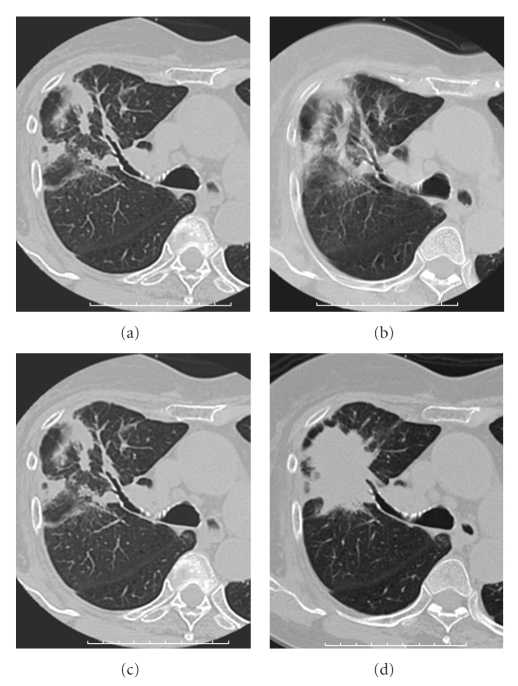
Serial CT images after SBRT. (a), (b), (c) CT images at 2, 6, and 9 months after SBRT show irregularly shaped patchy consolidation in the irradiated area. (d) CT images at 12 months after SBRT showing the appearance of mass-like consolidation in the irradiated area.
